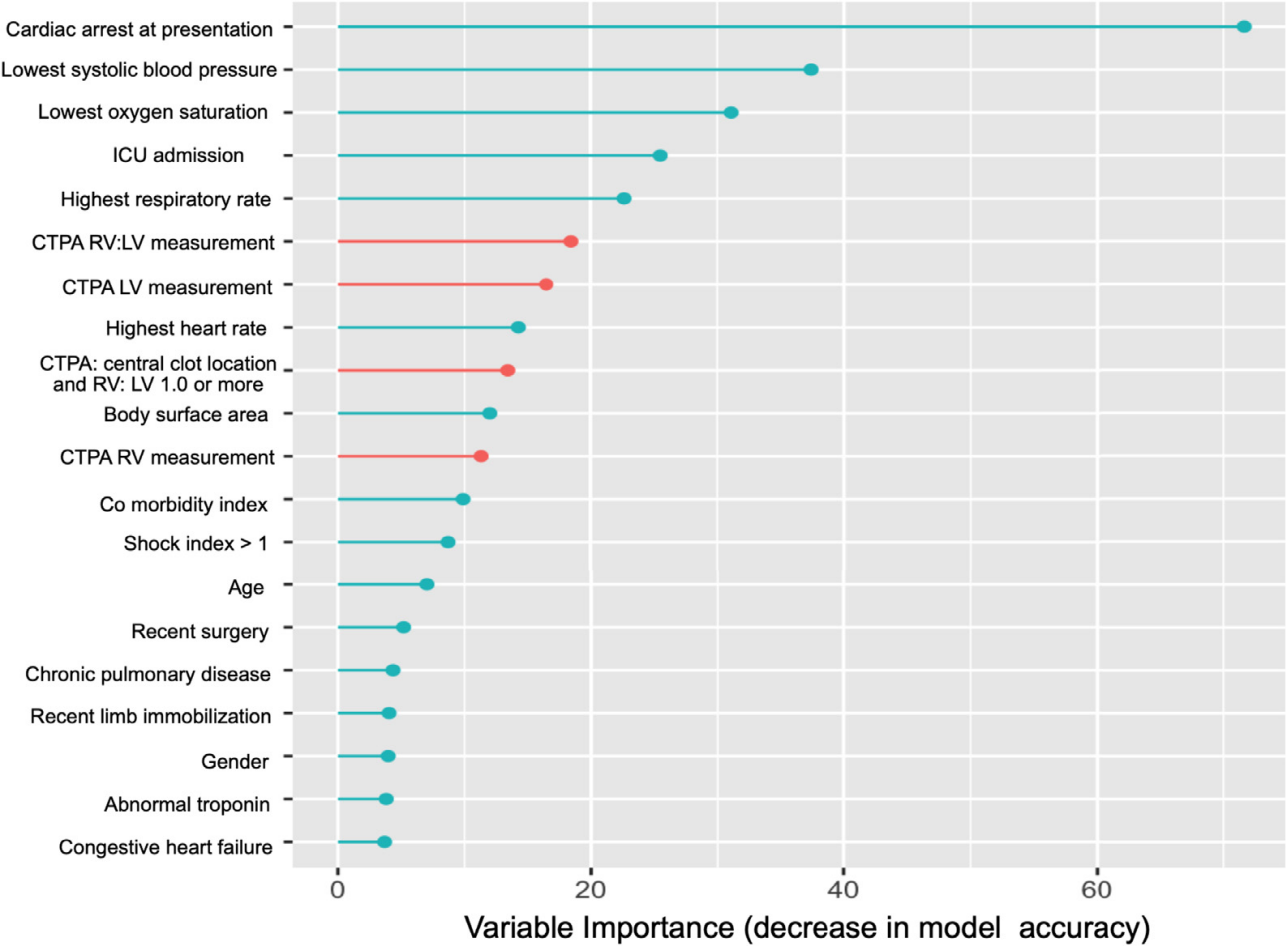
Random forest variable importance plot for predicting clinical deterioration. *CTPA*, computed tomography pulmonary angiography; *LV*, left ventricle; *RV*, right ventricle; *ICU*, intensive care unit.
